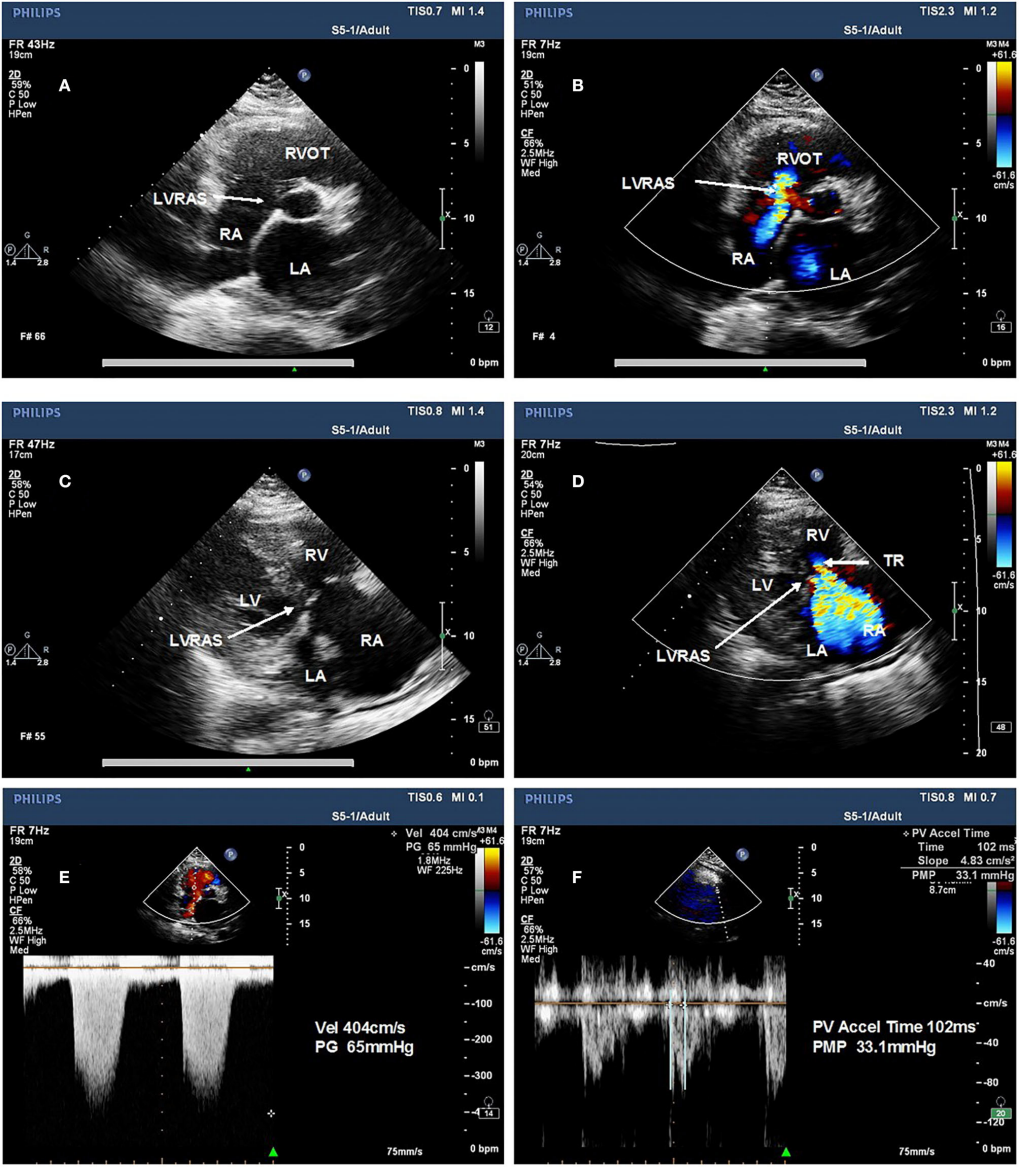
Representative examples of left ventricular-to-right atrial shunt characteristic from a representative patient on TTE imaging. (A) small defect in the atrioventricular portion of the interventricular septum in the parasternal short-axis view. (B) Left ventricular-to-right atrial shunt in the parasternal short-axis view. (C) small defect in the atrioventricular portion of the interventricular septum in the parasternal four chamber view. (D) Left ventricular-to-right atrial shunt in the parasternal four-chamber view. (E) the pressure gradient of the TR is higher and difference from mean pulmonary pressures and absence of the sign of pulmonary hypertension. (F) mean pulmonary pressures of the same patient. LA, left atrium; LV, left ventricle; RA, right atrium; RV, right ventricle.
